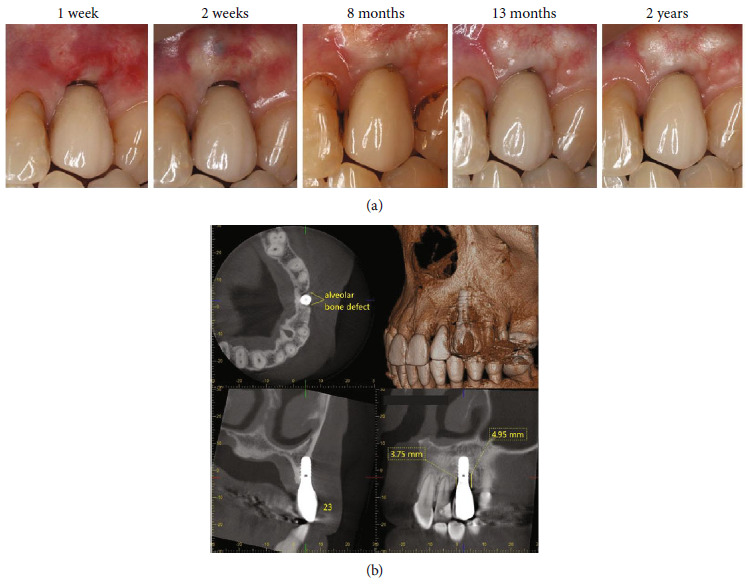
(a) Clinical appearance at the follow-up periods after surgery. (b) CBCT radiographs at 2 years after surgery demonstrating the remaining intrabony defect depth 3.75 mm at the mesial and 4.95 mm at the distal surfaces of the implant. The width of the intrabony defect was narrower than the pretreatment.
